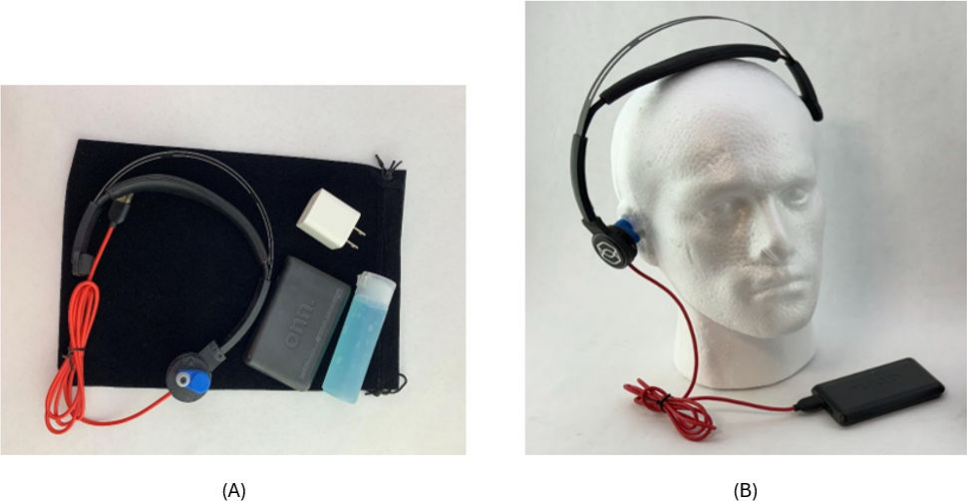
(A) The ZenBud headset, powerpack, power brick, and bottle of gel. The ultrasound transducer is located in the round earpiece on the right side of the headset. (B) The ZenBud device as depicted properly placed on a model human head.
